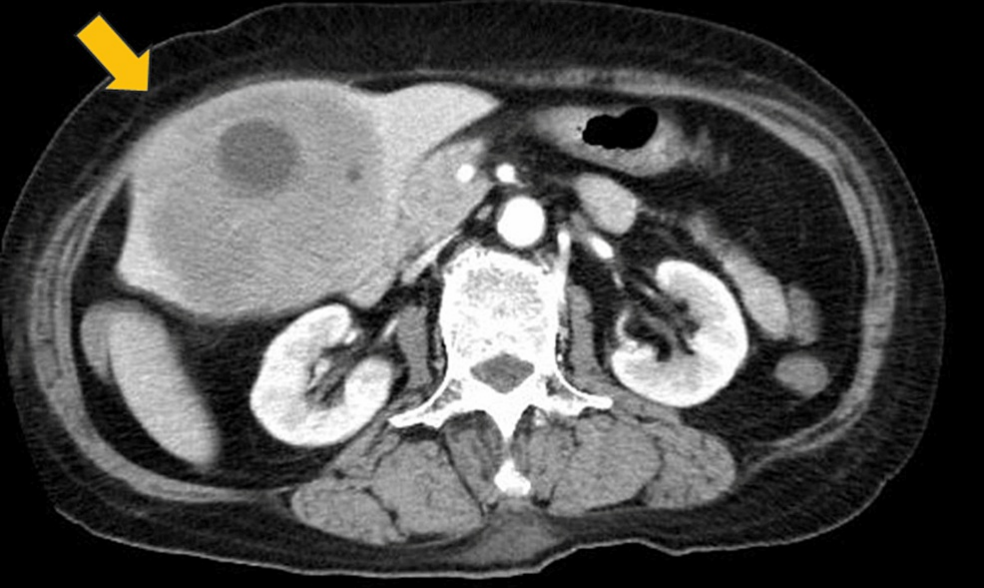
Contrast-enhanced computed tomography scan showed a huge hypoenhancing tumor in the left liver with a bulging, exophytic contour (arrow).
